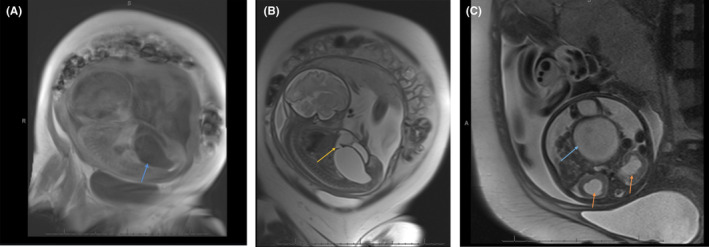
(A) Abdominal cyst appreciated in maternal/fetal MRI (arrow). This finding prompted further imaging with fetal MRI. (B) Abdominal cyst with fistula connecting to another cystic structure (arrow). The presence of a cystic abdominal mass raised concern for Bardet–Biedl Syndrome during prenatal care, and prompted the family to deliver at our institution where NICU and pediatric urology services were available. (C) Abdominal cystic mass (blue arrow) with bilateral hydronephrosis (red arrows). In addition to the abdominal cystic mass, hydronephrosis secondary to urinary tract compression is commonly seen in patients with Bardet–Biedl Syndrome
